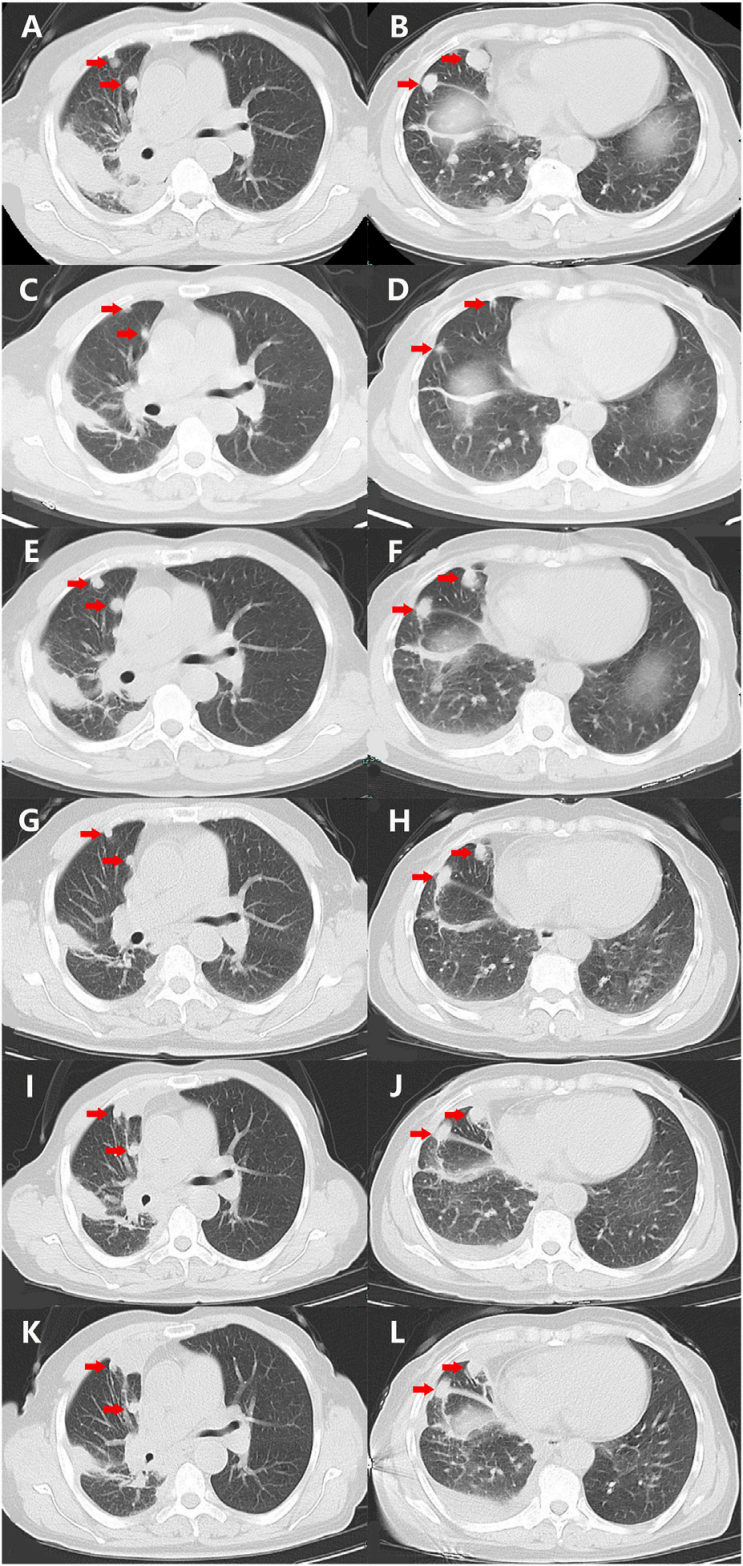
Variations of the primary and metastatic lesions in lung detected by chest CT scans during the treatment (red arrowheads). (A,B) Chest CT scans showed the primary and metastatic lesions (red arrowheads) in the lungs on 20 November 2021. (C,D) Chest CT scans showed the primary and metastatic lesions (red arrowheads) in the lungs on 5 January 2022. (E,F) Chest CT scans showed the primary and metastatic lesions (red arrowheads) in the lungs on 15 March 2022. (G,H) Chest CT scans showed the primary and metastatic lesions (red arrowheads) in the lungs on 12 April 2022. (I,J) Chest CT scans showed the primary and metastatic lesions (red arrowheads) in the lungs on 29 June 2022. (K,L) Chest CT scans showed the primary and metastatic lesions (red arrowheads) in the lungs on 25 July 2022. Abbreviations: CT, computerized tomography.
